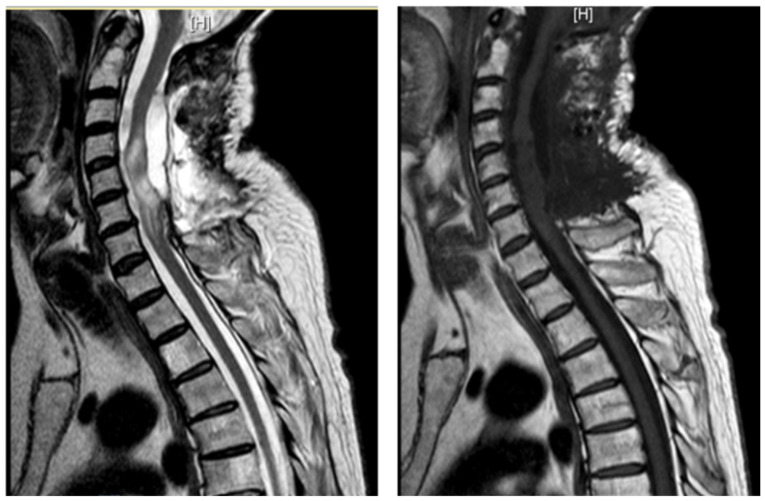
Case 2: pre- and post-operative MRI showing reduction in the myelopathy area.
